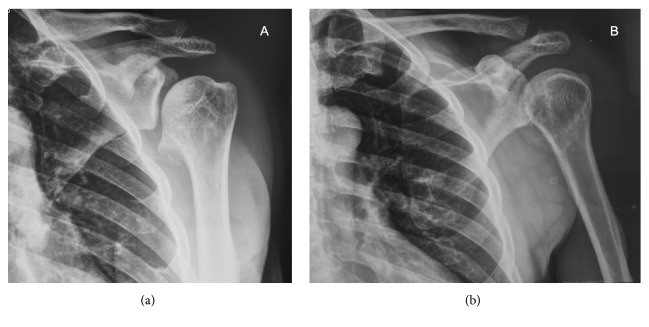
Radiographs of the left shoulder demonstrated abnormal morphology of the left humerus head, characterized by a large anteromedial exostosis arising from the epiphysis of this bone. The initial diagnosis was DEH. In addition, slight proximal migration of the distal part of the left clavicle was noted (Rockwood and Neer grade 2 AC dislocation), with no signs of AC degenerative disease. (a) True AP view and (b) AP view with maximal internal rotation.
